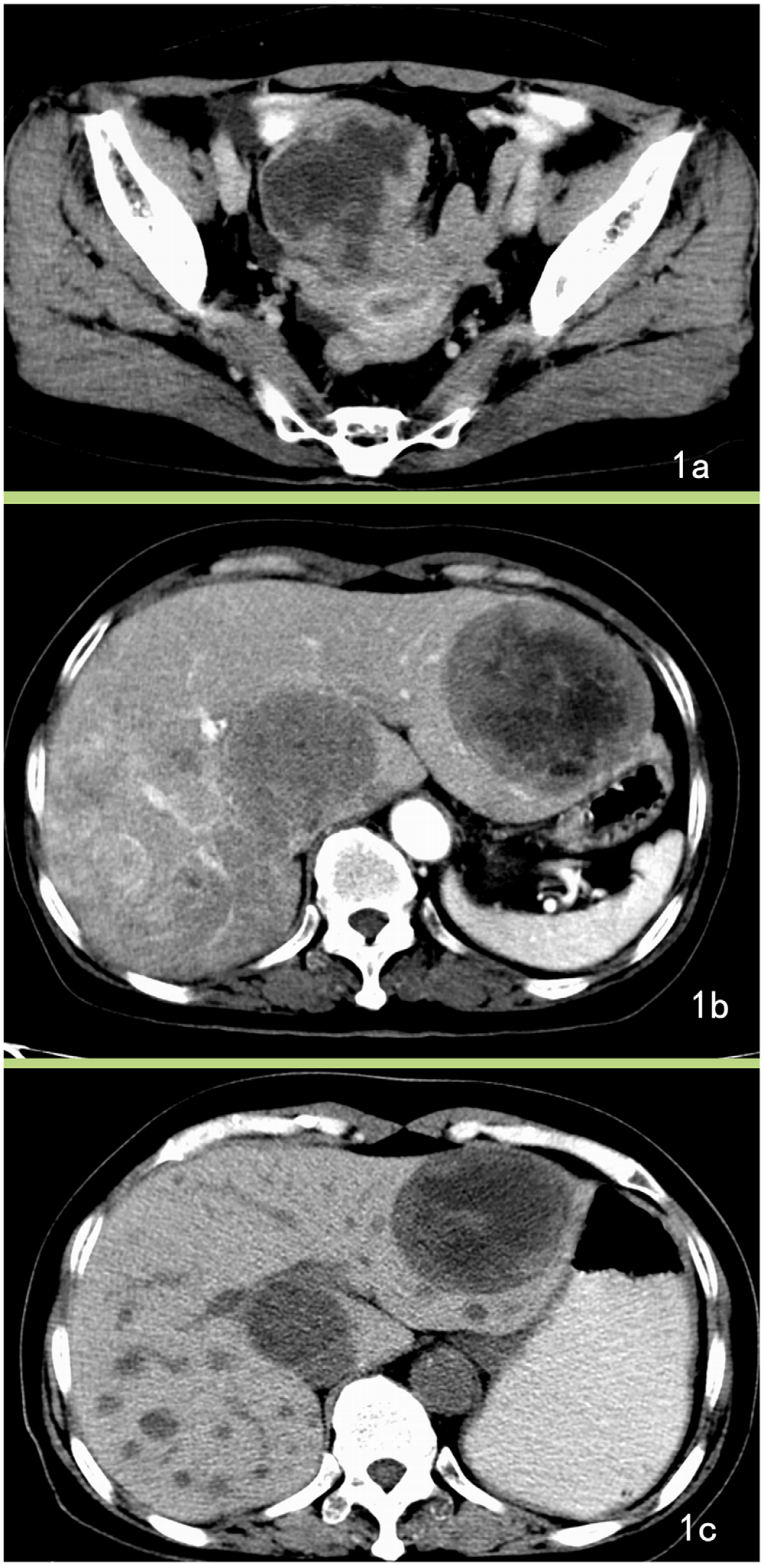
Jejunum GISTs with liver metastasis. A 64-year-old female with jejunum GISTs with liver metastasis presented with clinical abdominal pain for 6 months, and ultrasound examination detected liver masses for 1 week. A. Enhanced CT image reveals a lobular mass with severe necrosis and periphery enhancement. B. The axial section of the liver shows low-density lesions in the liver with slight enhancement. The patient was diagnosed with a malignant stromal tumor and hepatic metastasis. C. After treatment with Gleevec, the neoplasm became cystic and reduced in size.
